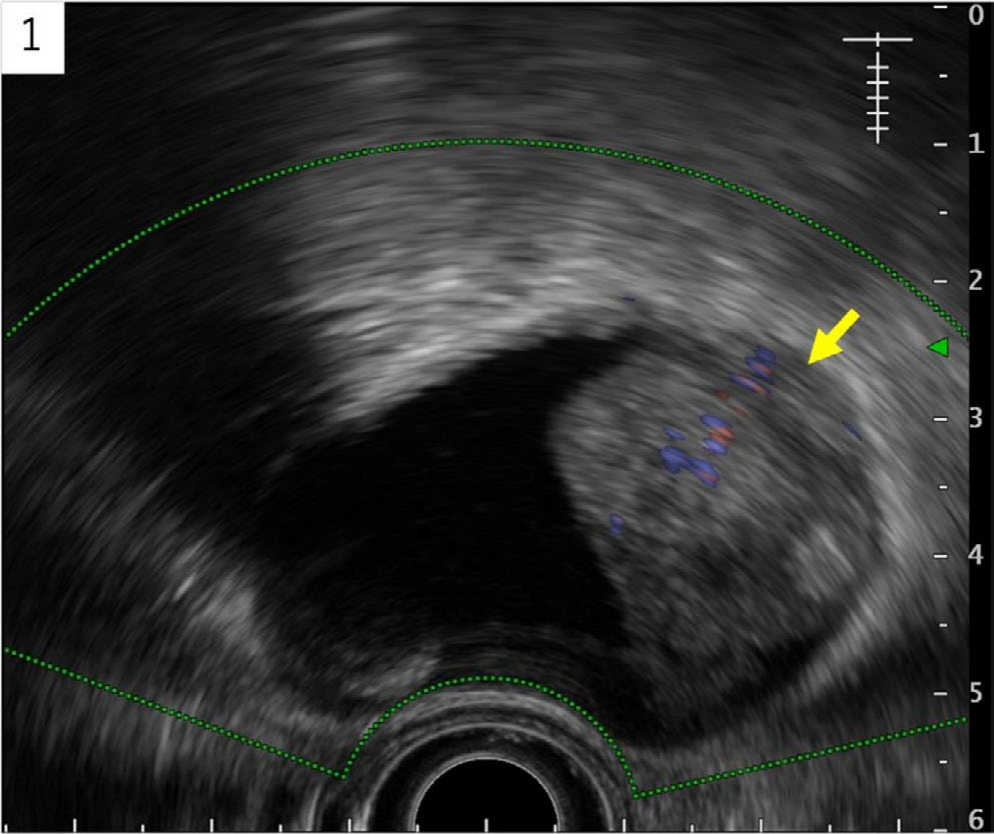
Endoscopic ultrasonography. A 28 mm irregularly‐shaped inhomogeneous, hyperechoic semi‐pedunculated lesion in the funds of gallbladder, and a blood flow signal on the inside by Doppler ultrasonography.
